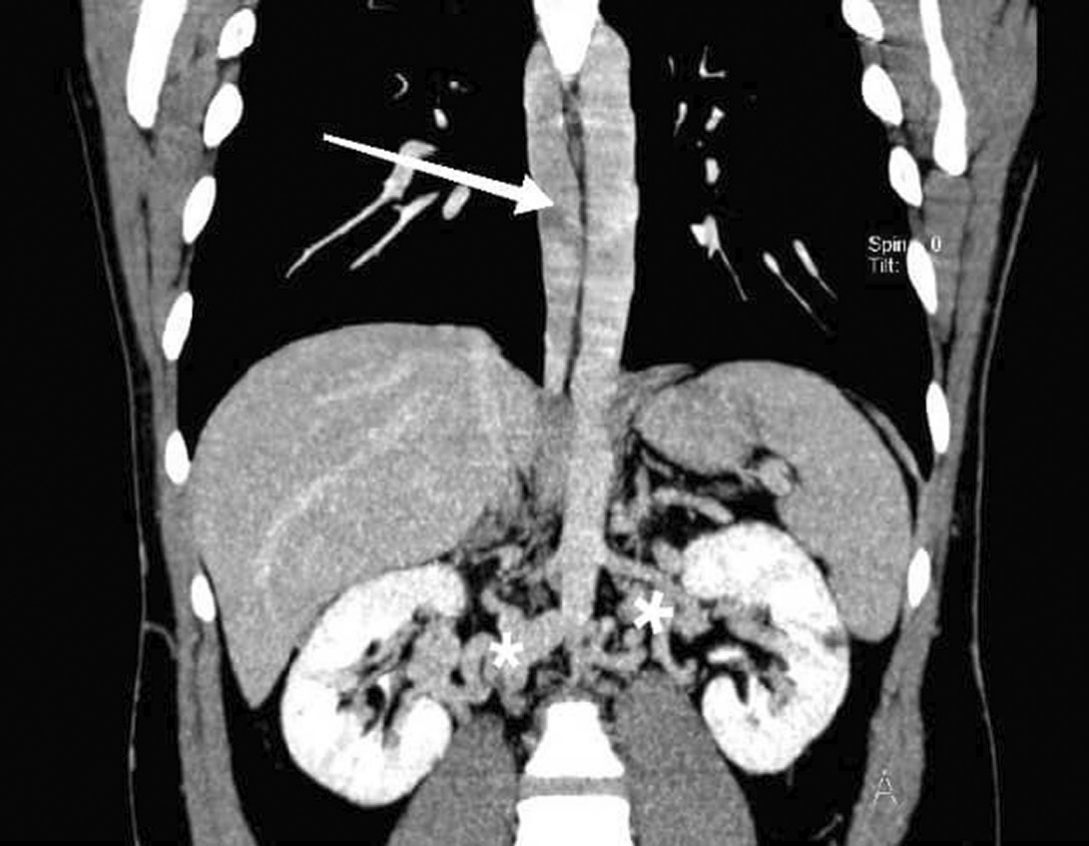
Coronal maximum intensity projection computed tomography image shows dilated azygos vein (arrow) in right side of vertebral column and multiple venous collaterals in hilum of kidneys (asterisks)
